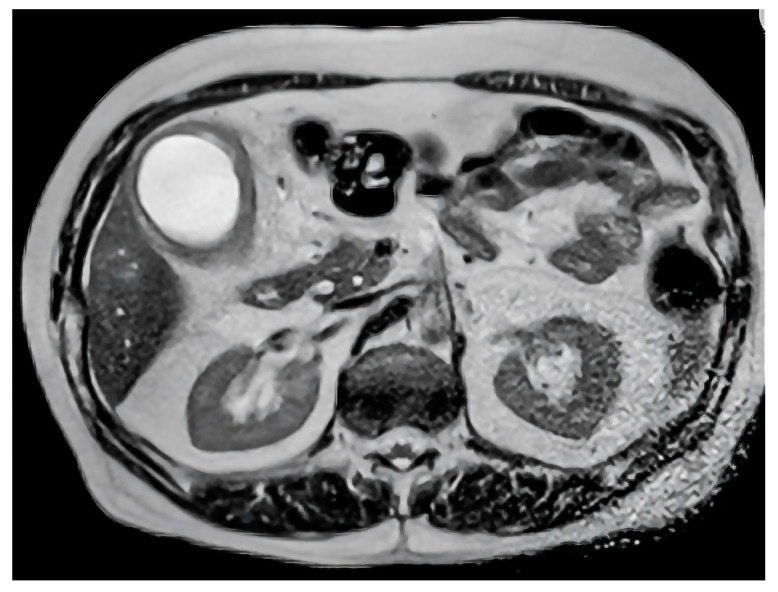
Axial T2-weighted MRI scan demonstrating gallbladder wall thickening and intramural nodules, characteristic of xanthogranulomatous cholecystitis.
